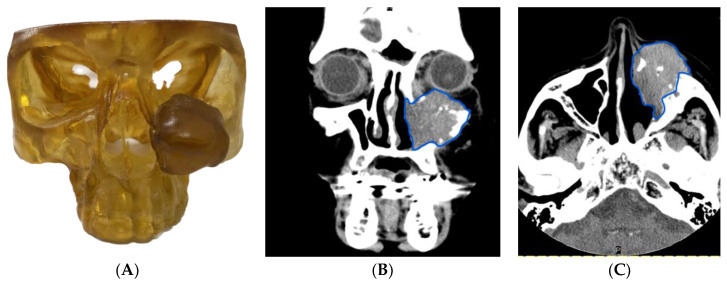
(A) 3DM of the patient’s tumor demonstrating the extent of local invasion, (B) coronal CT image demonstrating the extent of inferior tumor invasion (blue outline highlighting tumor), and (C) axial MRI image highlighting the posterior and medial extent of tumor invasion.
